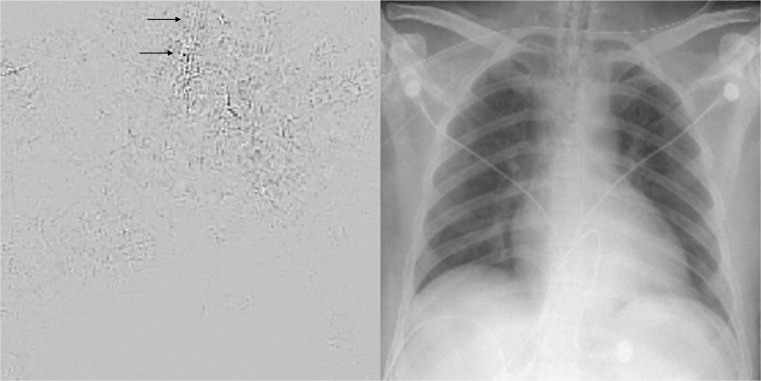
Saliency map on the *left* with corresponding radiograph on the *right*, where an ET tube is present. The saliency map highlights parts of the images that contribute most to the prediction class. The *black arrows* points to the location of the ET tube, which has a visible contribution to the prediction label. However, there are other parts of the image, including edges of ribs and the aortic knob that also contribute to the score for this image. While the network had high accuracy, there is still room for improvement
